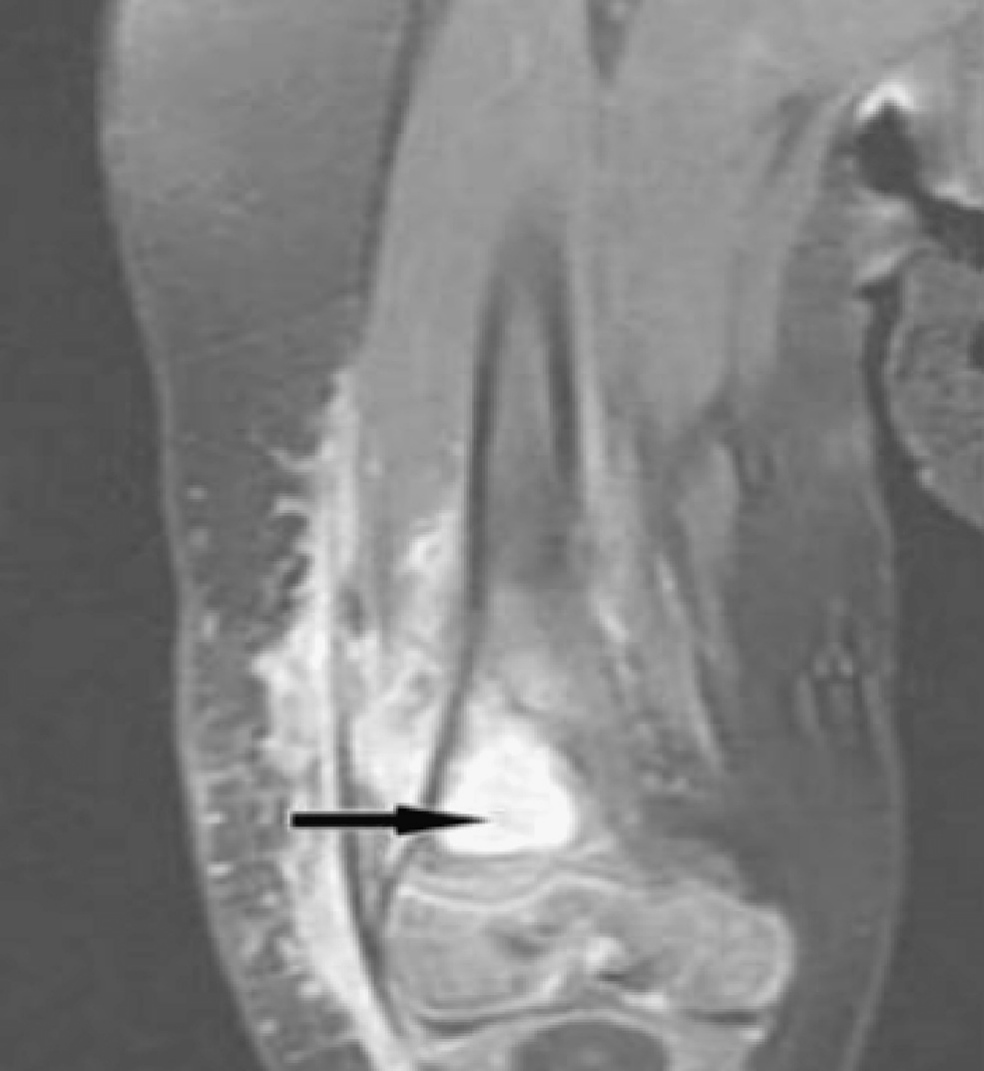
MRI showing a lytic lesion in the distal right femur with soft tissue extension
